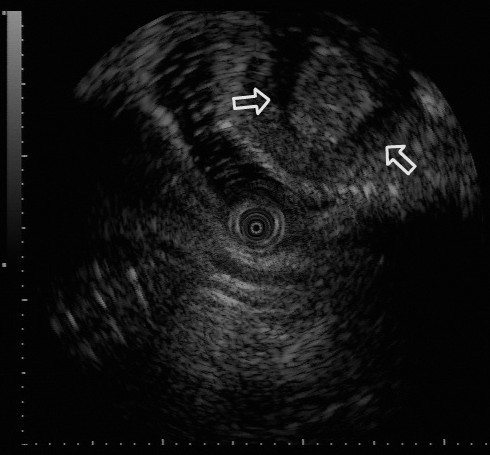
Endoscopic ultrasound examination. Endoscopic ultrasound examination of the tumor revealed a hypo-echoic layer corresponding to the muscularis propria and a hyper-echoic layer corresponding to fat tissue.
